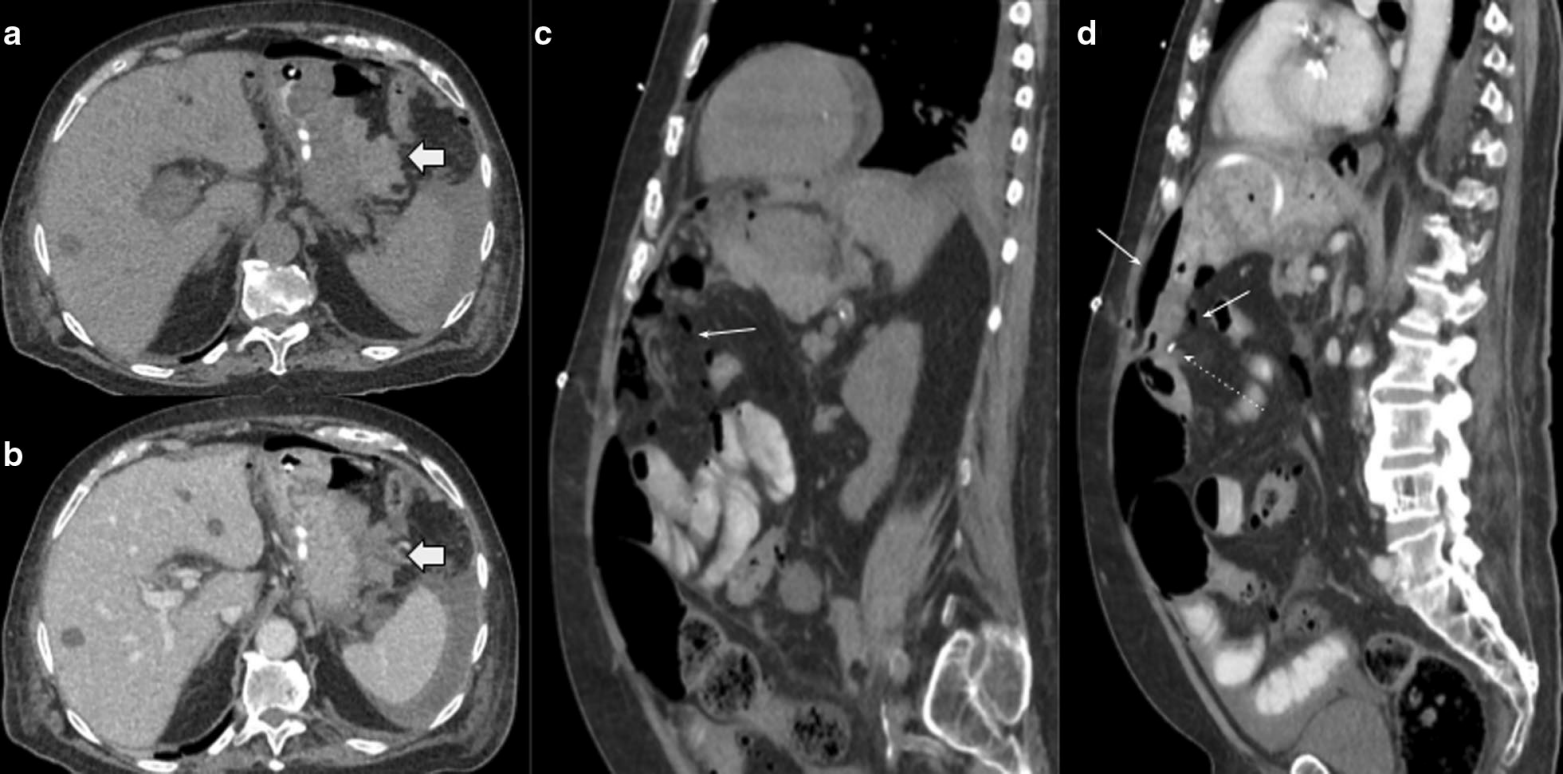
Gastrojejunostomy dehiscence. 87-year-old male patient with complicated early postoperative duodenopancreatectomy with Y de Roux and gastrojejunostomy due to pancreatic adenocarcinoma. Axial and sagittal non-contrast (a–c) and portal venous phase (b–d) CT scan. Shows a blood collection (big arrows in a and b) associated with pneumoperitoneum (thin arrows in c and d) adjacent to the gastroenteric anastomosis (dotted arrow in d). Urgent laparoscopy confirmed gastroenteric anastomosis dehiscence
